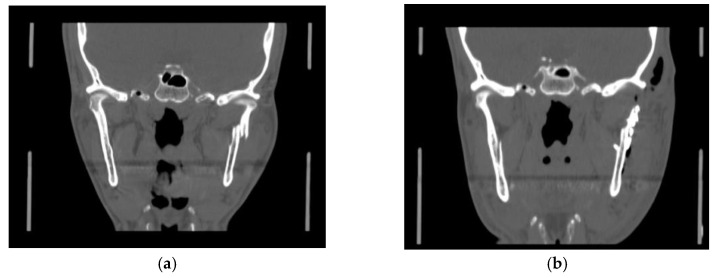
(a) Pre- operatory coronal CT scan; (b) post-operatory coronal CT scan of patient #2.
